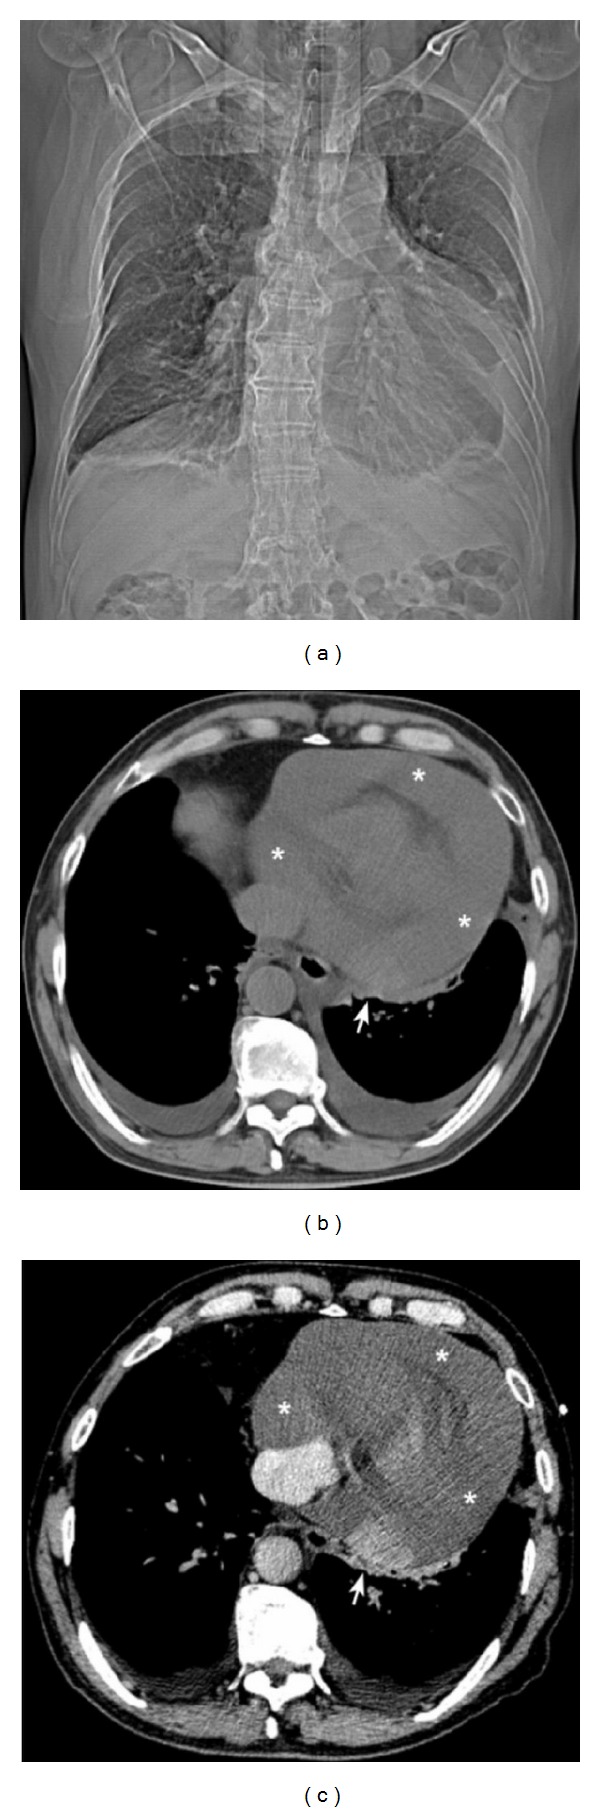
(a) Computed tomography (CT) scanogram/scout shows enlarged cardiac silhouette with blunting of the left costophrenic sulcus. (b) Nonenhanced chest CT image reveals a nearly isoattenuating nodular mass arising from the left posterior pericardial wall (arrow) with a large circumferential pericardial effusion (asterisks) and bilateral pleural effusions without signs of cardiac tamponade. (c) Contrast-enhanced chest CT scan shows a well-defined, enhancing nodule (arrow).
